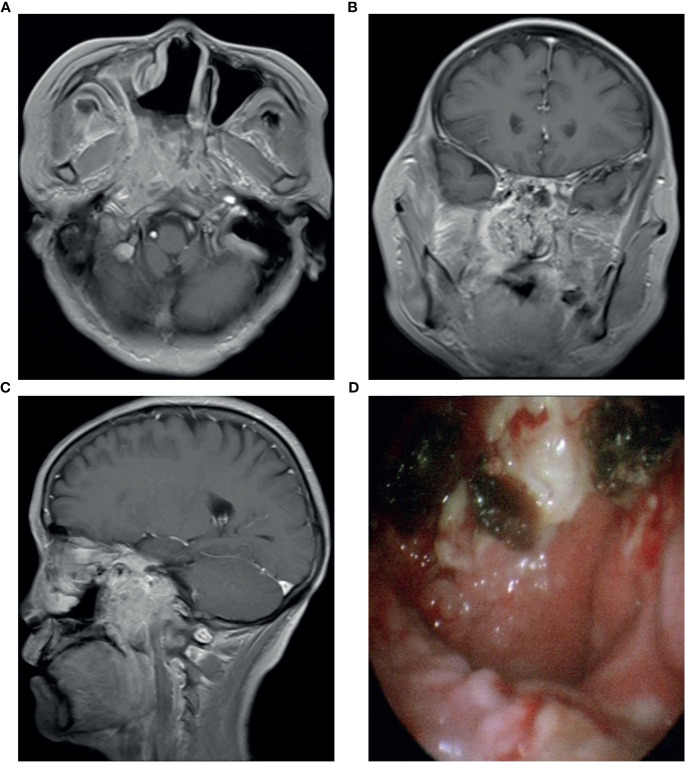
Contrast enhancement T1-weighted MR imaging showed that the tumor invaded the parapharyngeal space and nasopharynx (A–C); (D) Electronic nasopharyngoscope showed new organization in the nasopharynx.
